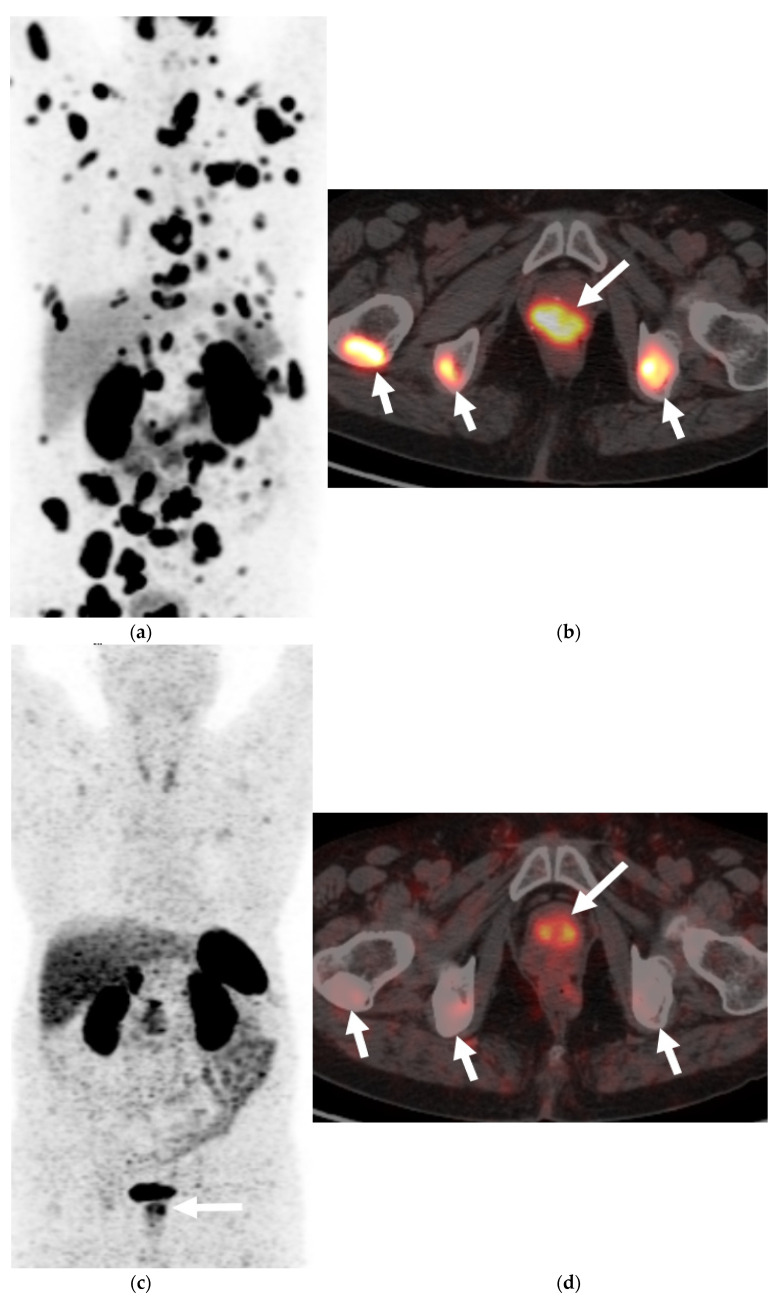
An elderly patient with metastatic neuroendocrine prostate cancer. Coronal maximum intensity projection (MIP) PET image (a) and axial color fused image of 68Ga DOTANOC PET-CT (b) show intensely hypermetabolic prostatic mass (long arrow) consistent with neuroendocrine prostate cancer and hypermetabolic widespread bony metastases (short arrows). Post-treatment DOTANOC PET-CT following 4 cycles of PRRT therapy shows marked positive treatment response. There was near complete metabolic response in the bony metastases, as seen on the coronal MIP PET image (c) with minimal residual metabolic activity corresponding to some sclerotic bony metastases on axial color fused PET-CT image (d). There is also interval decrease in the extent and degree of hypermetabolic activity in the prostatic malignancy with residual activity suspicious for residual viable malignancy. Image courtesy: Divya Yadav, M.D.
